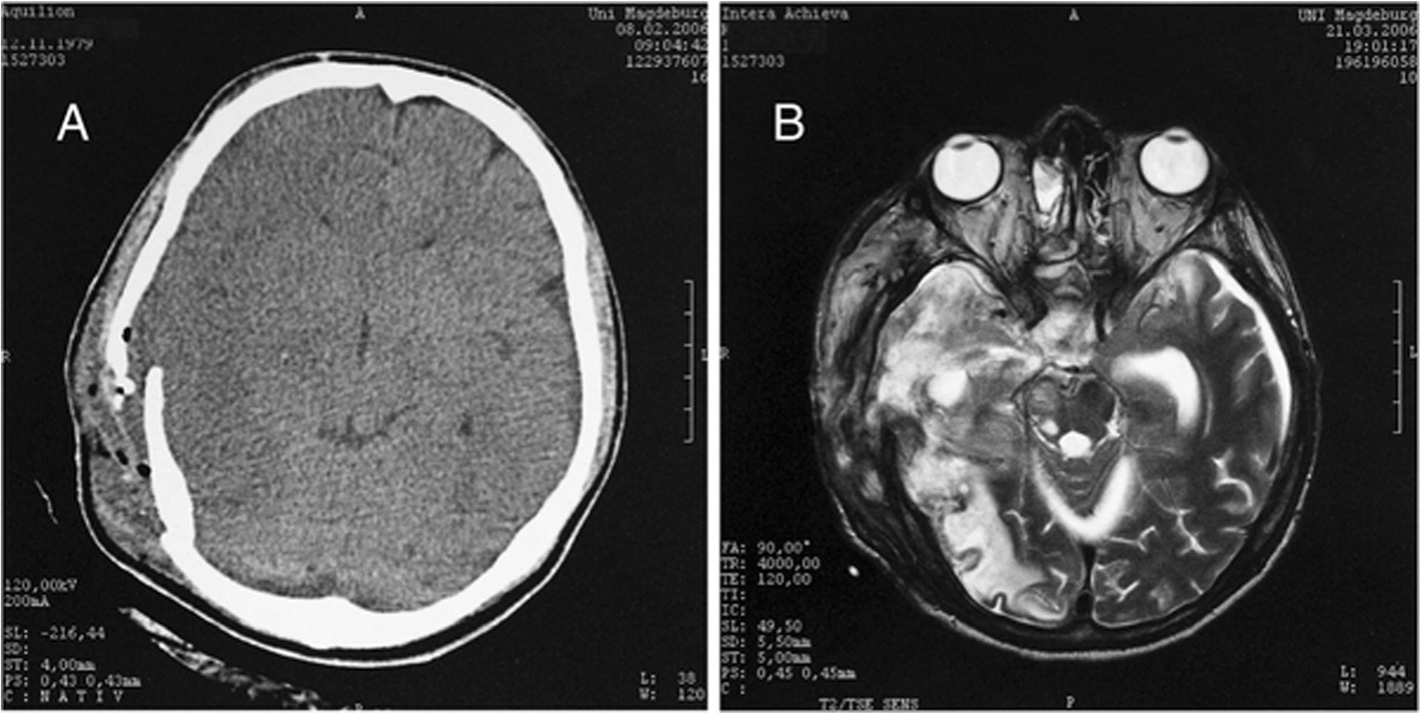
26 y/o patient with traumatic brain injury. A. CT scan only shows impression fracture and mild swelling. B. MRI shows severe contusion of right temporal lobe and bilateral mesencephalic lesions causing coma.
